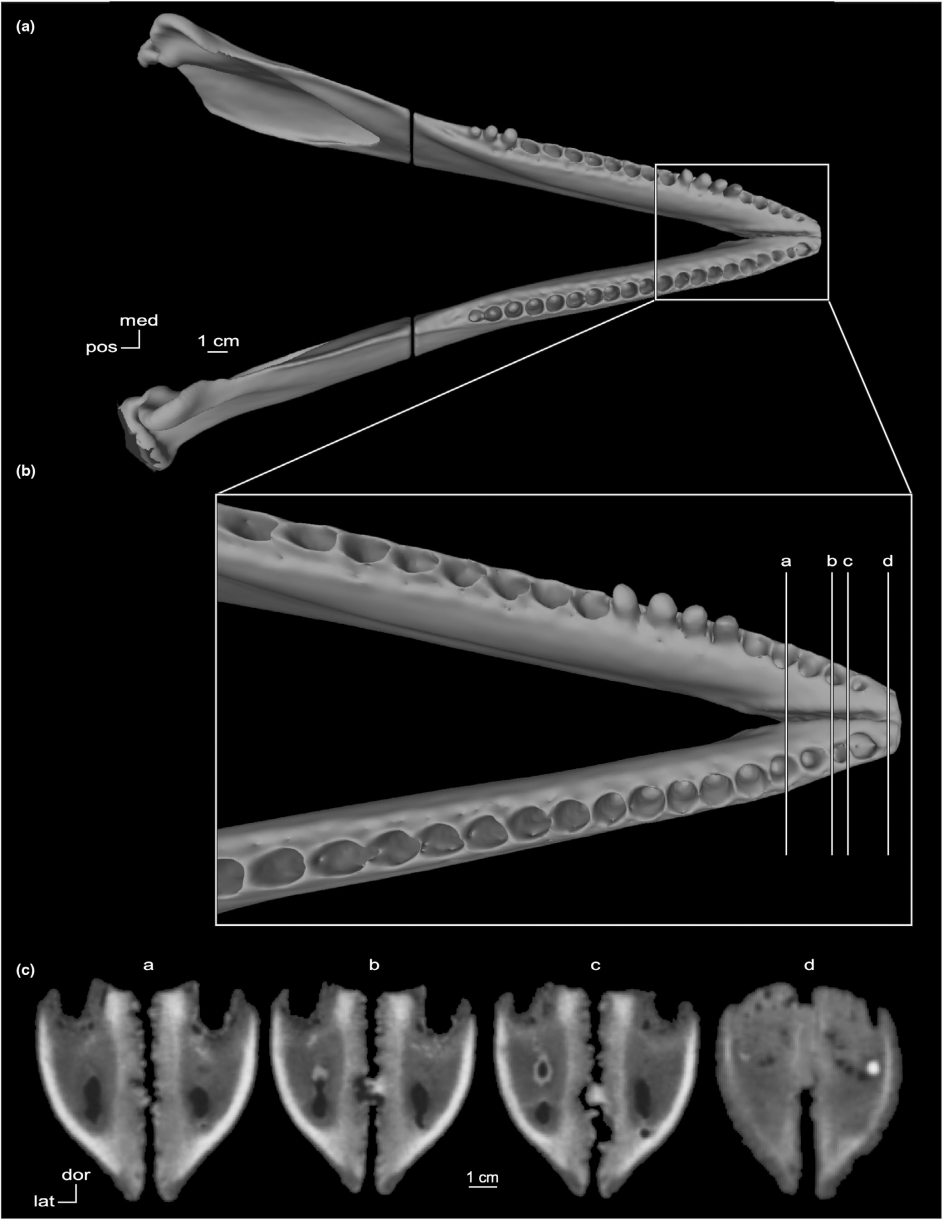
Mandibles of *Tursiops truncatus* (SDSNH 21212). (a) Dorsal view. (b) Close‐up of the symphysis in dorsal view showing the level of CT cross‐sectional slices (a–d). (c) CT coronal cross‐sectional slices (a–d).
